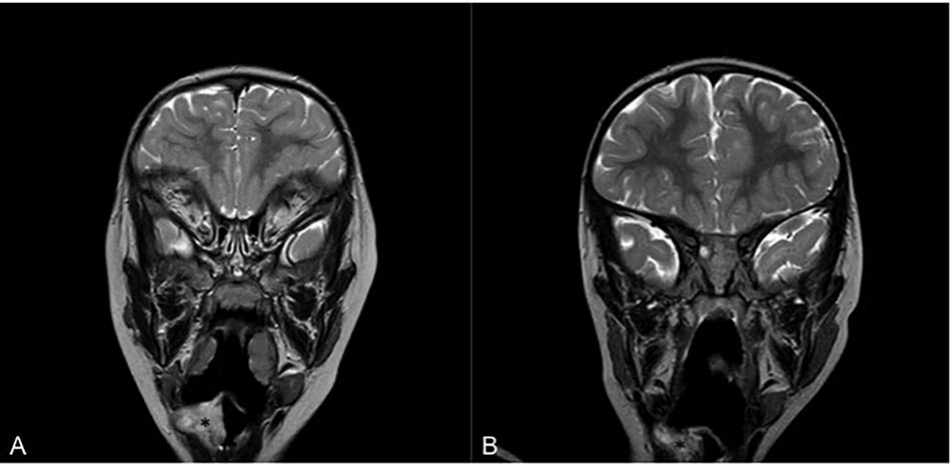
Magnetic resonance imaging (MRI) of head and neck with contrast in sagittal view on admission (A) and at 16 months follow-up (B). Asterisk denotes area of interest.
